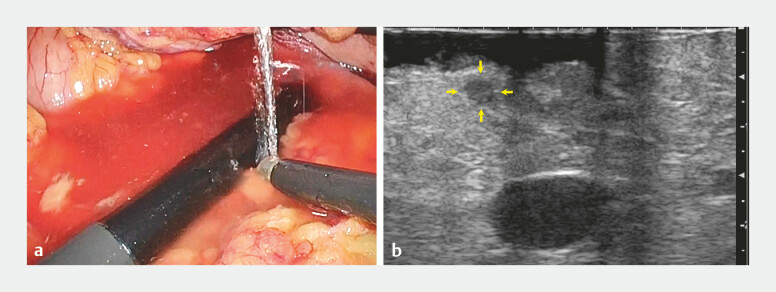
Detecting the small pancreatic tumor using laparoscopic ultrasound by collaboration between surgeons and endoscopists.
